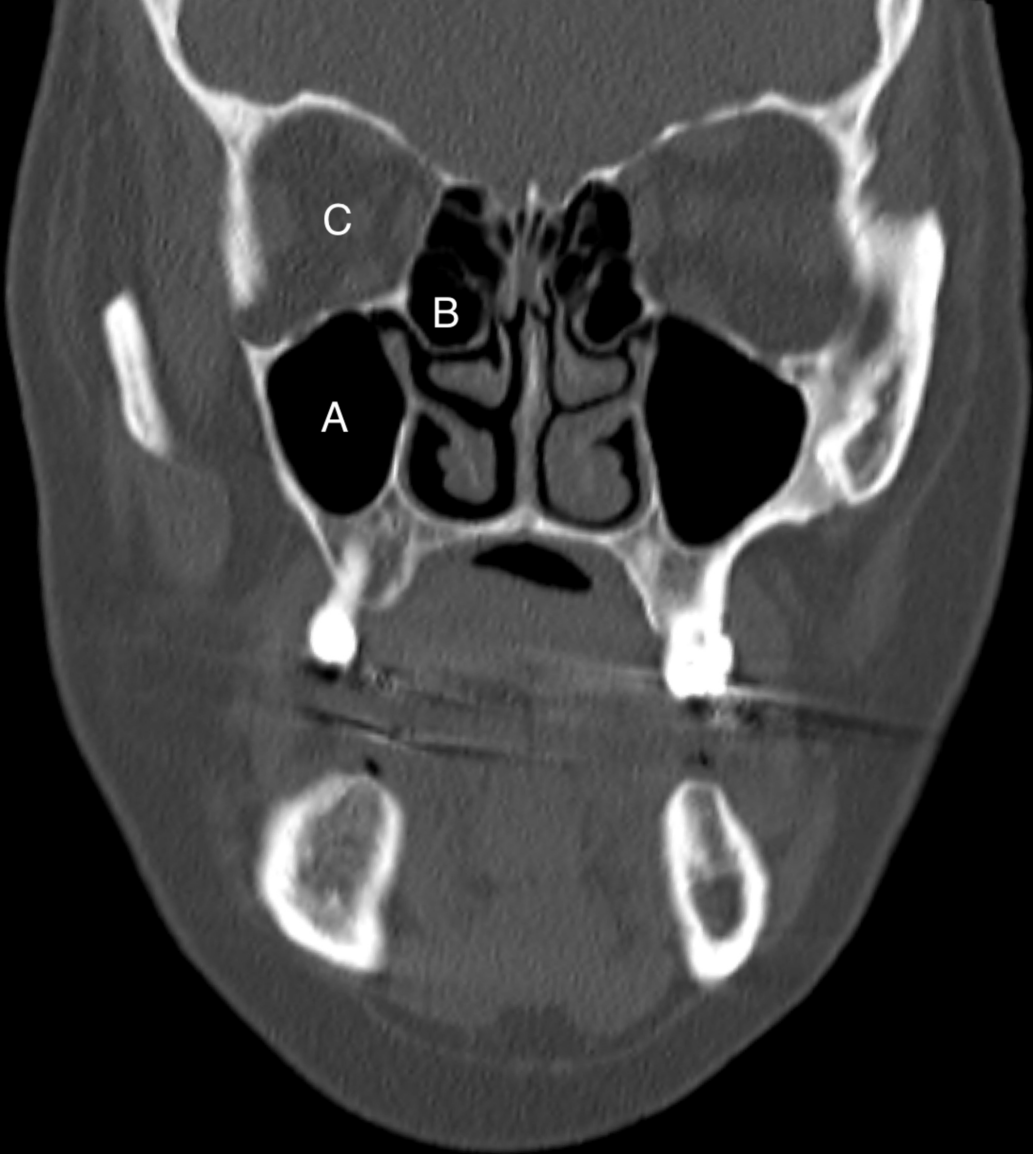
A coronal CT image of the paranasal sinuses, orbits and brain of this patient. There are no signs of sinusitis: no mucosal thickening or opacification in any of the visible sinuses, including the right maxillary sinus (A) and the right ethmoid sinus (B). The orbit (C) does not demonstrate any intra-orbital pathology.
